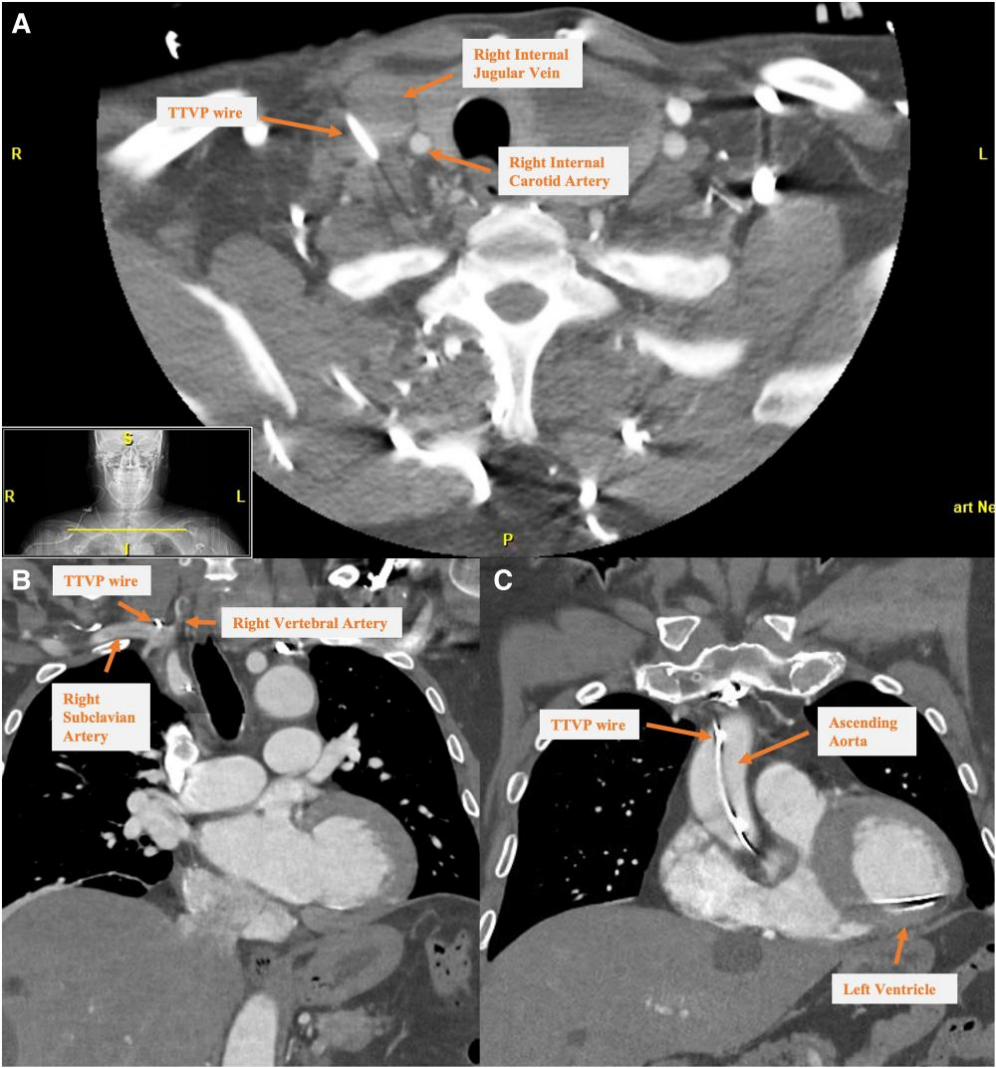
(*A*) CT: temporary transvenous pacing wire lateral to right IJ vein. (*B*, *C*) Wire entering superior vena cava, subclavian artery, brachiocephalic artery, and ascending aorta, with lead in left ventricle.
